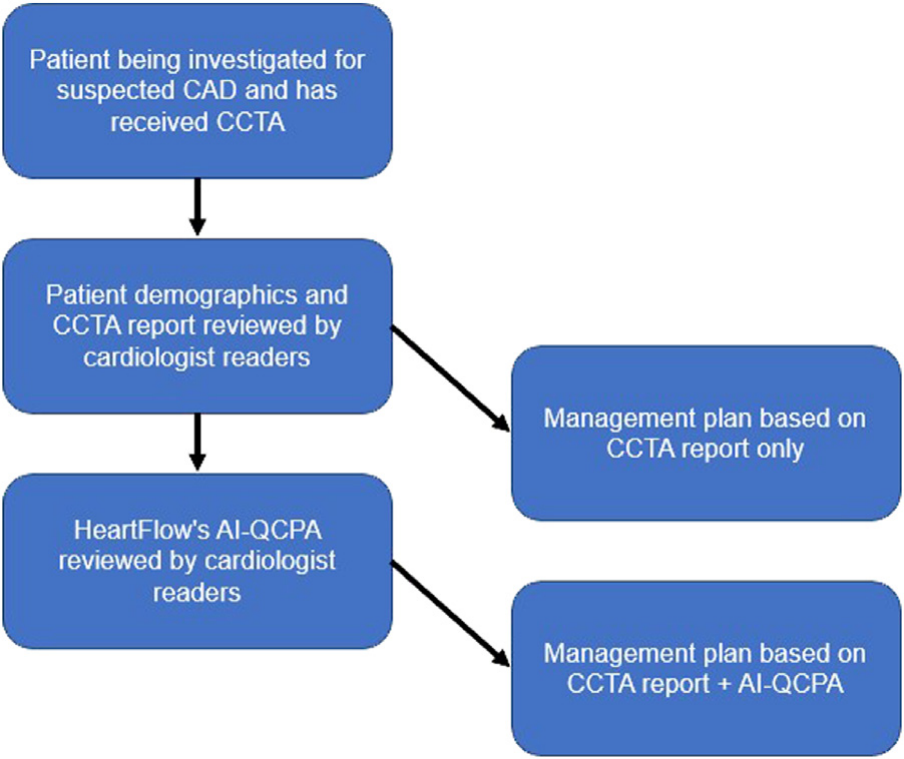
Study design. AI-QCPA, artificial intelligence quantified coronary plaque analysis; CAD, coronary artery disease; CCTA, coronary computed tomographic angiography.
